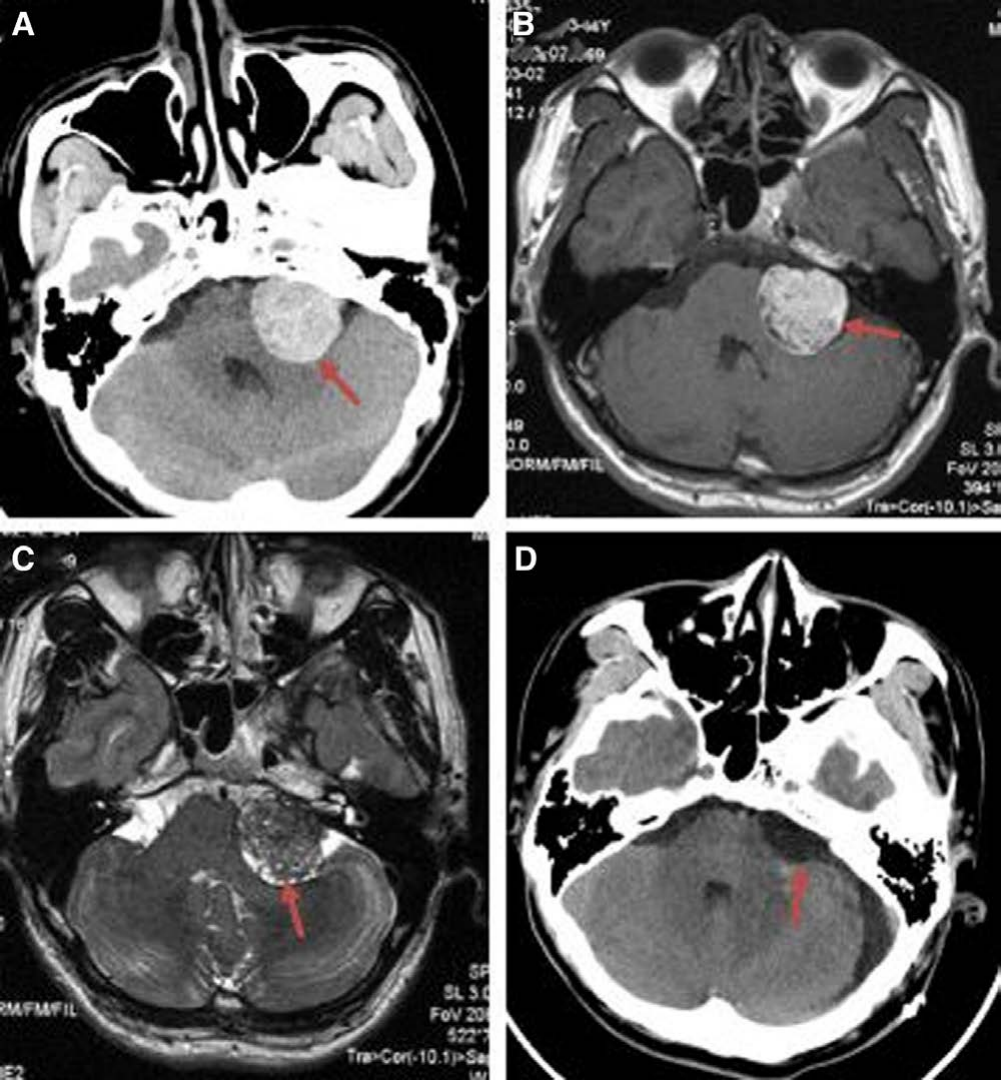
Preoperative and postoperative imaging of a 34-year-old male patient with left cerebellopontine angle melanoma. (A) Preoperative axial CT showed a high-density round lesion (red arrow). (B) Preoperative axial T1WI showed a high-signal round lesion (red arrow). (C) Preoperative axial T2WI showed a low-signal round lesion (red arrow). (D) Axial CT at 1 day after surgery showed total tumor resection (red arrow). WI = weighted image.
